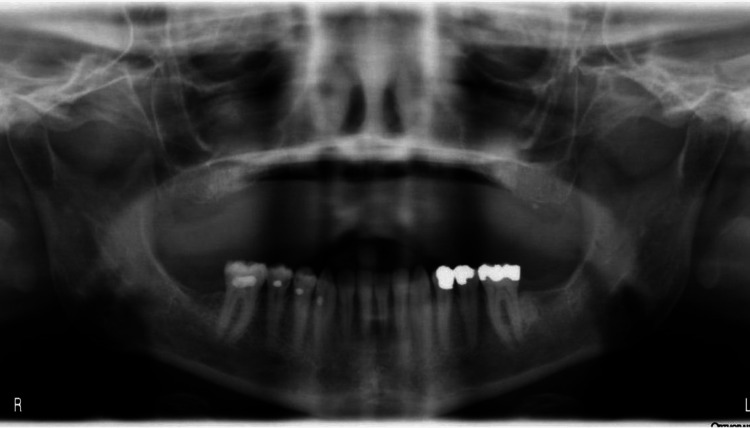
Conventional panoramic radiograph
